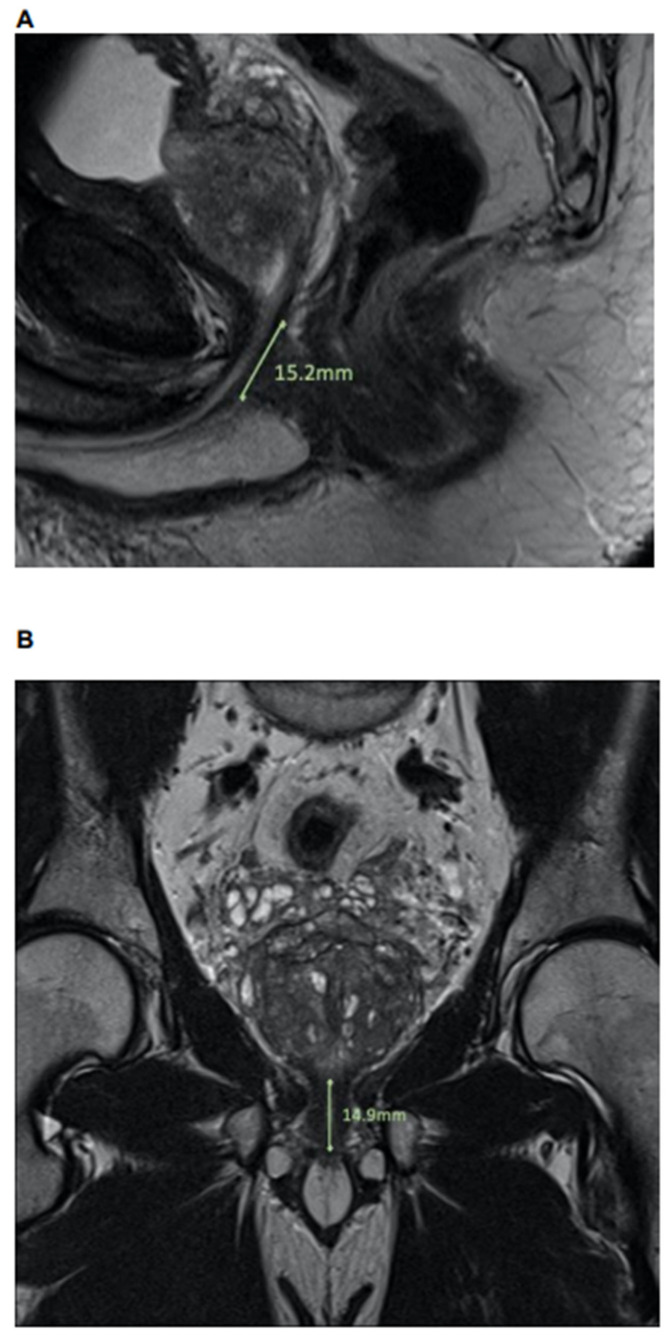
Urethral sphincter length measurements in (A) sagittal and (B) coronal dimensions in a multiparametric MRI prior to radical prostatectomy.
